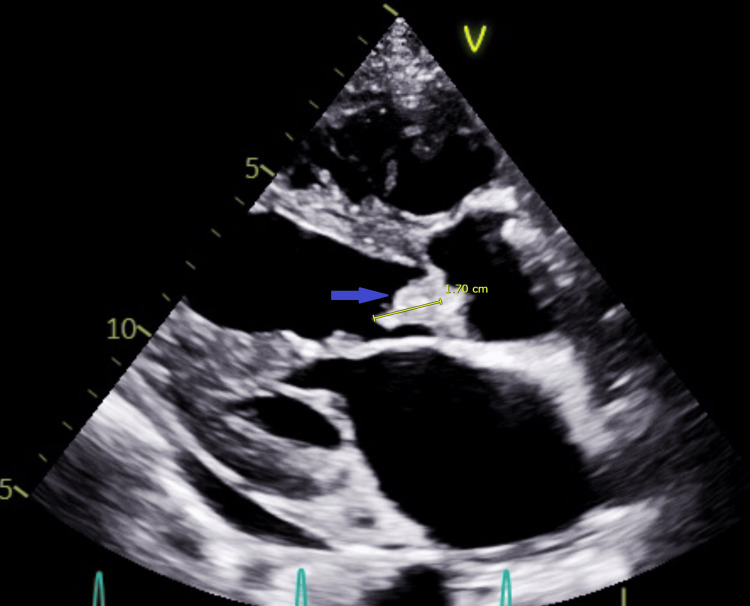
Transthoracic echocardiogram parasternal long-axis view showing a 17 mm vegetation on the aortic valve (blue arrow)
